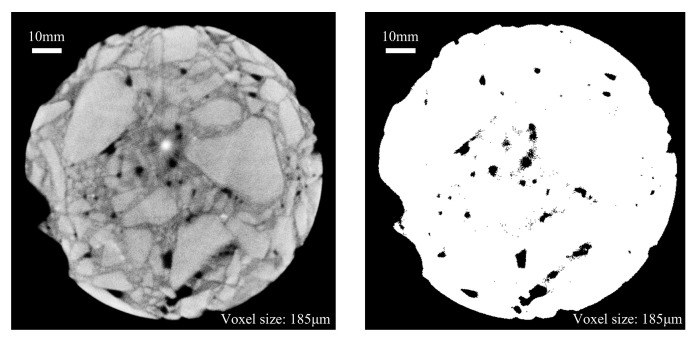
CT images before and after image processing.
